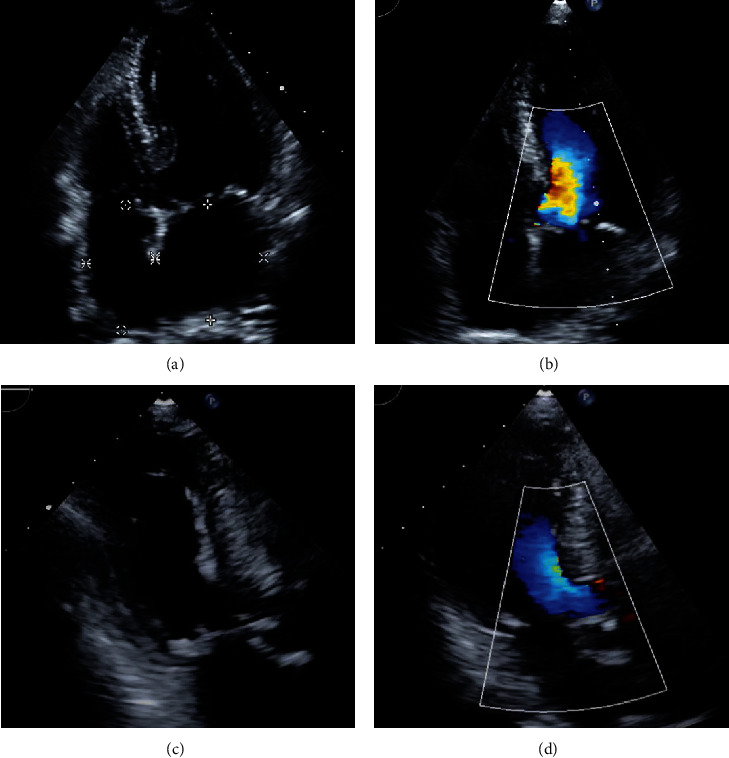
The preoperative echocardiogram was showing no obstruction of the left ventricular outflow tract. (a) The apical four-chamber view. (b) The apical four-chamber view with flow mapping. (c) The apical three-chamber view. (d) The apical three-chamber view with flow mapping. A normal left ventricular contraction and mild aortic regurgitation was noted on the apical three-chamber view (Supplement video 1).
